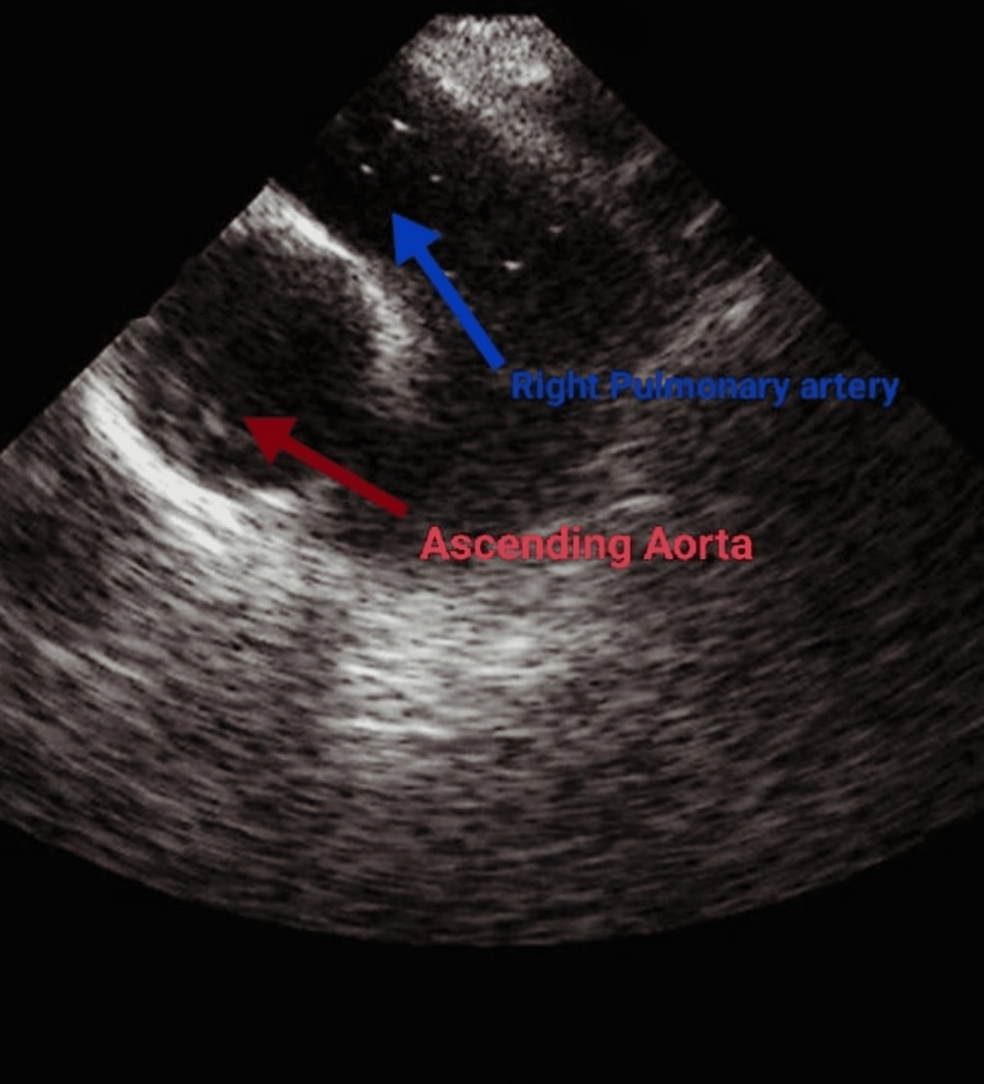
Transoesophageal echocardiography demonstrating air bubbles in the pulmonary artery.
